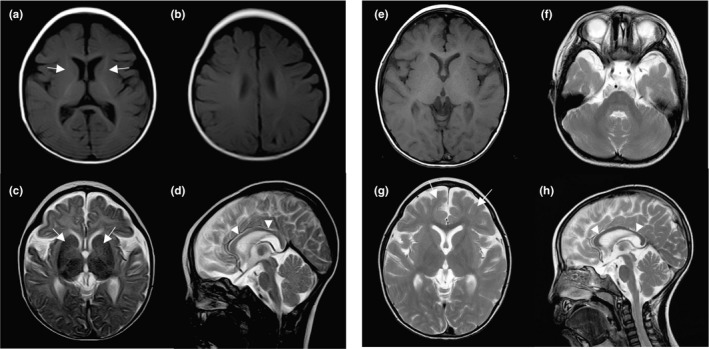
Brain magnetic resonance images of the patient at 7 months (a–d), and 3 years (e–h). T1‐weighted images (a, b and e), and T2‐weighted images (c, d, f, g and h). (a) The posterior limb of the internal capsule shows high intensity suggesting myelination, but the anterior limb of the internal capsule only partly shows high intensity (arrows). (b) The white matter of the centrum semiovale and the central sulcus circumference show slightly high intensity. (c) While the posterior limb of the internal capsule shows normal low intensity in T2‐weighted images, the anterior limb of the internal capsule shows high intensity, suggesting delayed myelination (arrows). (d) The corpus callosum is hypoplastic and shows isointensity, suggesting delayed myelination (arrowheads). (e) T1‐weighted image at 3 years shows high intensity in the subcortical and deep white matter, suggesting almost full myelination. (f) The white matter of the cerebellum still shows high intensity in T2‐weighted images. (g) Part of the subcortical white matter of the frontal lobes does not show low intensity (arrows), suggesting incomplete myelination in some parts. (h) The hypoplastic corpus callosum shows hypointensity, suggesting progress of myelination (arrowheads)
